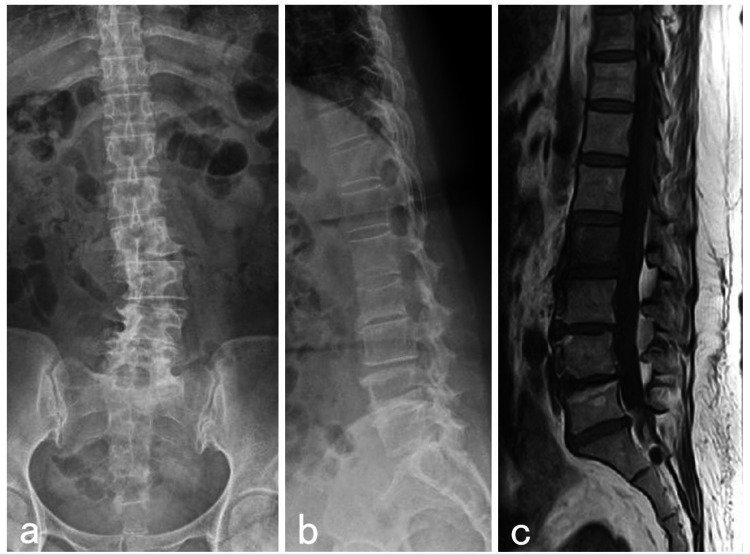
Second imaging examination Anterior-posterior radiograph shows a fracture of the inferior end plate of L3 vertebral body (a). Lateral radiograph shows wedge deformity of L3 vertebral body (b). The magnetic resonance imaging with a T1-weighted sagittal image reveals an expanded range of signal changes in the L3 vertebral body (c).
